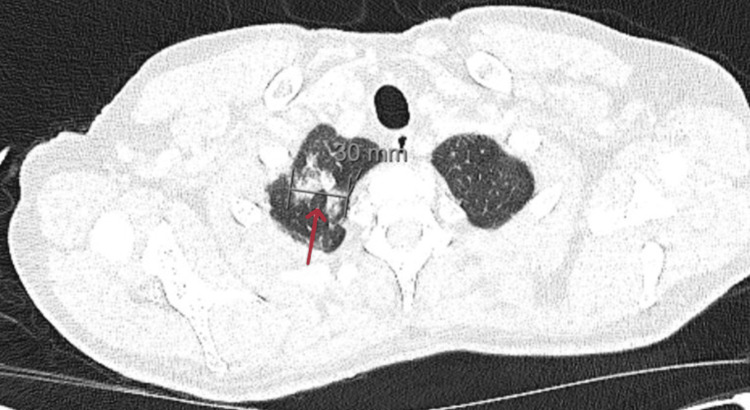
CT image showing focal ground glass changes right upper lobe in lung
